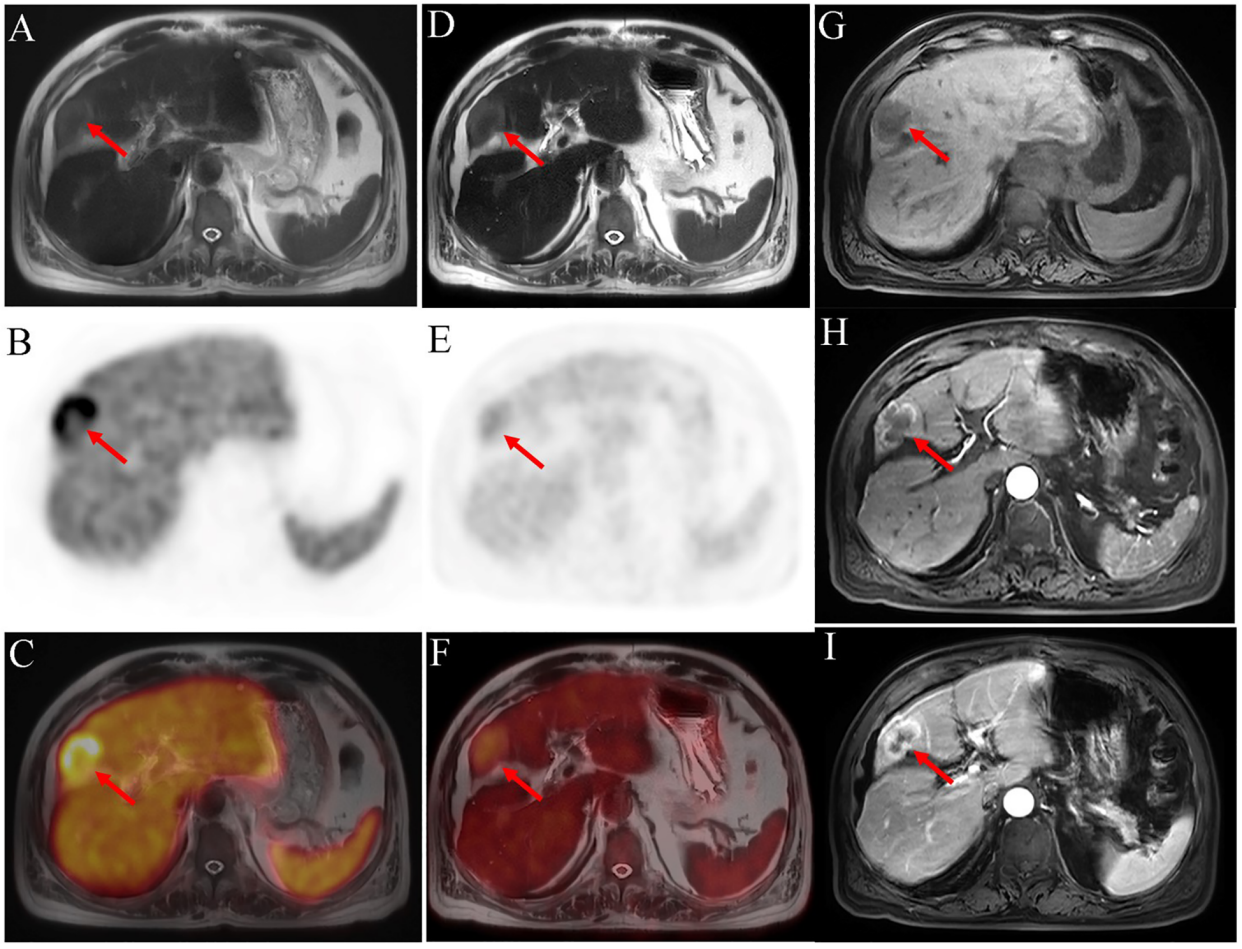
^18^F-PSMA-1007 PET/MRI [(A) axial T2WI-MRI; (B) axial PET and (C) fused axial PET/MRI] shows a high PSMA uptake mass in the liver (SUVmax=18.76). In ^18^F-FDG PET/MRI imaging [(D) axial T2WI-MRI; (E) axial PET and (F) fused axial PET/MRI], mild uptake is seen. Contrast-enhanced MRI of the abdomen [(G) T1WI-MRI; (H) arterial phase T1WI-MRI and (I) portal phase T1WI-MRI] reveals a significantly enhanced margin of mass in arterial phase, with further enhancement in the portal phase. The center of the mass is a necrotic area with no enhancement or radiotracer uptake. Postoperative pathology indicates a moderate to poor differential cholangiocarcinoma. The red arrow shows the liver lesion.
